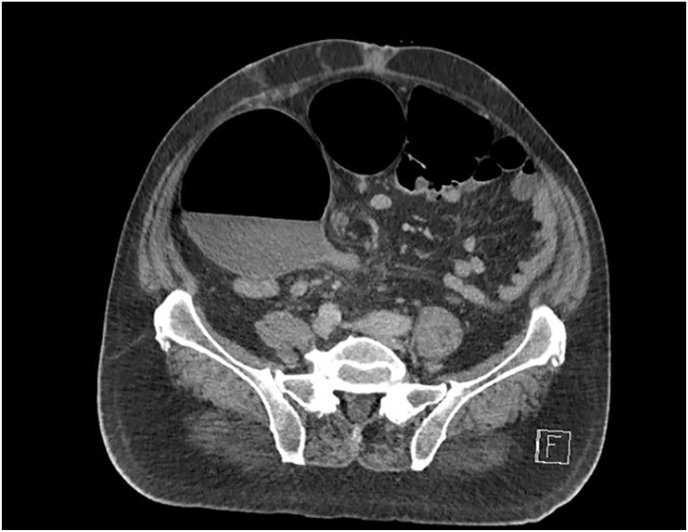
Sigmoid Volvulus Axial CT abdomen/pelvis.
